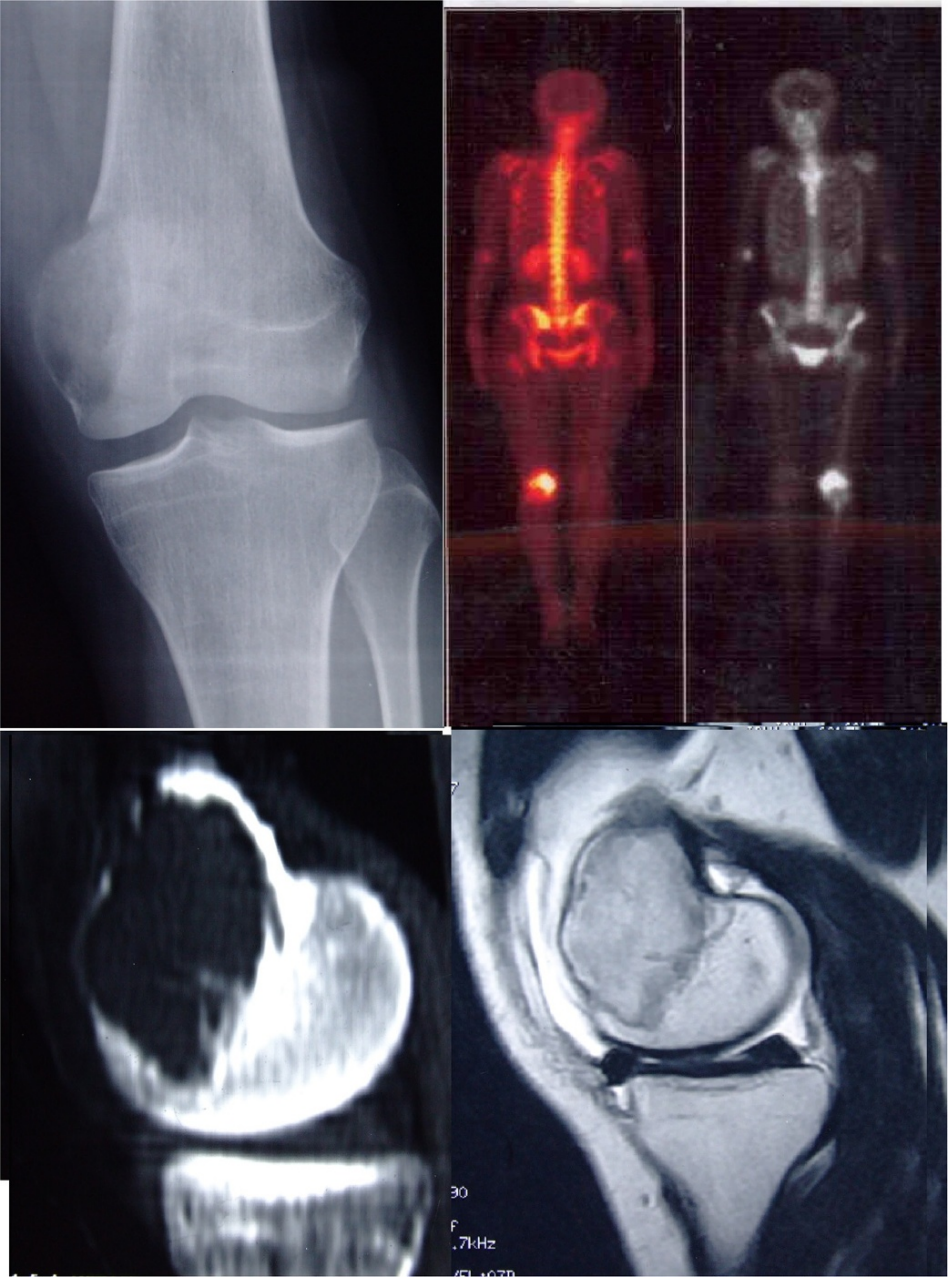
A 22-year-old female patient came to the orthopedic clinic complaining of continuous pain of left knee, X-ray,CT, MRI and bone scan tests found a sub-articular lucent lesion with clear boundaries on the left distal femoral bone.
